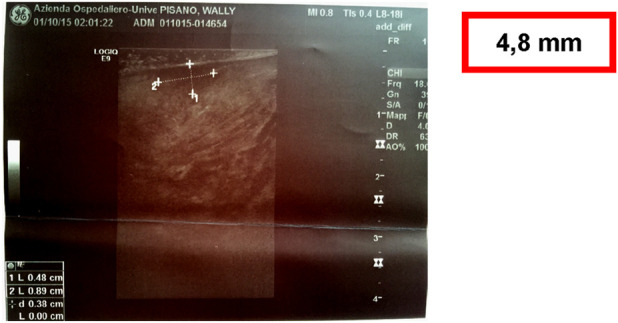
Intraoral high definition ultrasonography examination of the lesion showed an hypoechoic lesion measuring 4, 8 mm × 8.9 mm.
